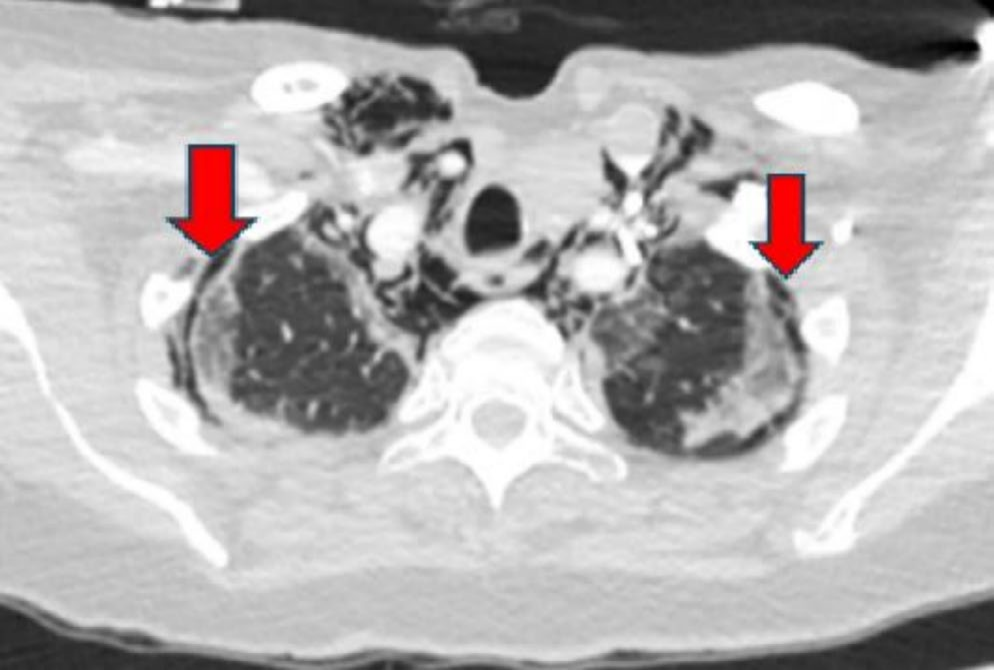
Chest scan demonstrating significant bilateral apical pneumothoraces. The red arrows are pointing to the areas of pneumothoraces on the CT chest scan.
